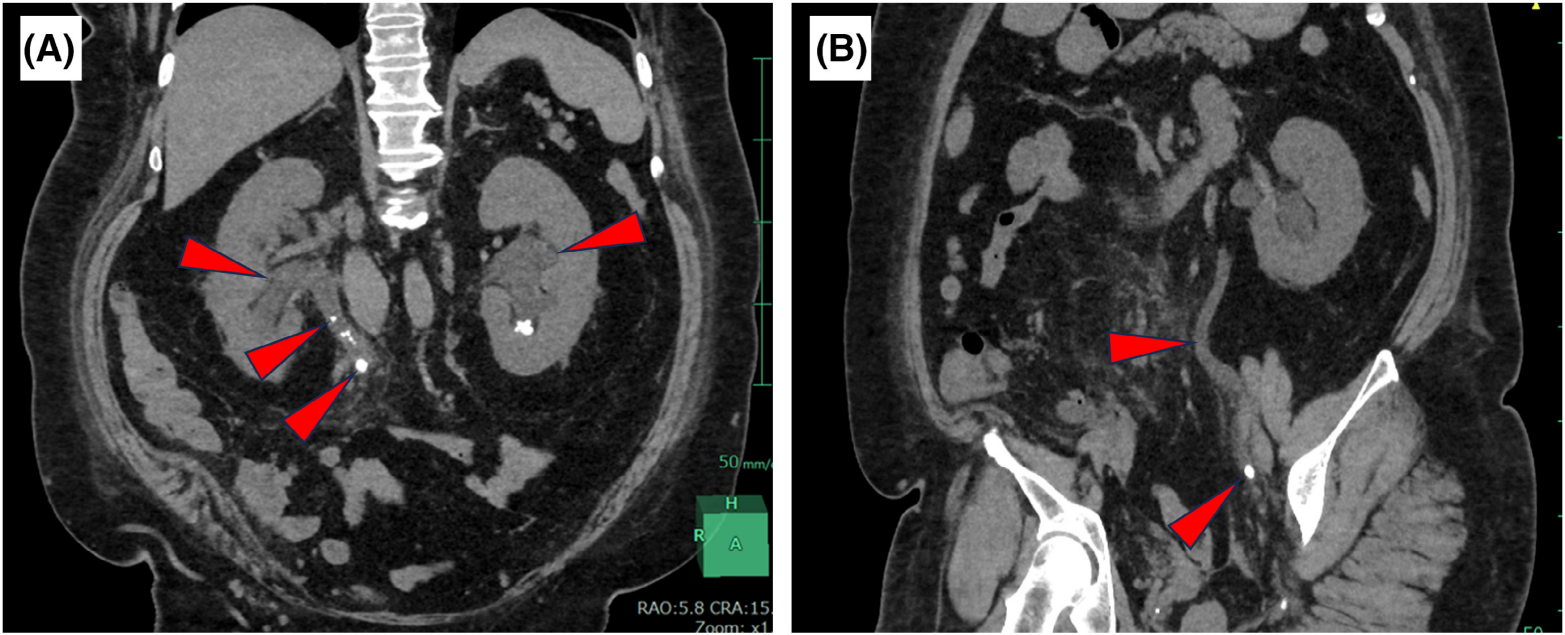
Computed tomography. (A) Coronal view showing bilateral hydronephrosis and right hydroureter and ureterolithiasis (red arrowheads). (B) Coronal view showing left hydroureter and ureterolithiasis (red arrowheads).
